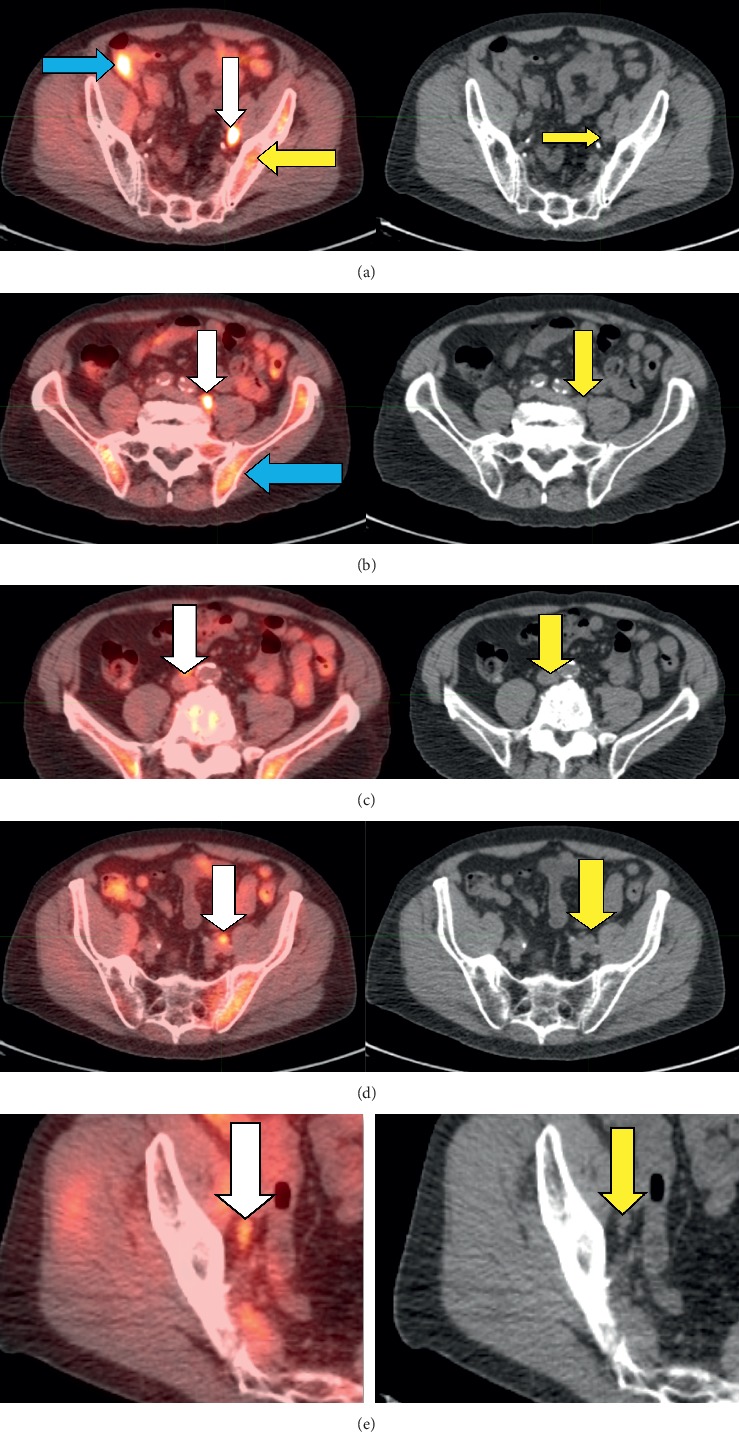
Images of a 68-year-old male who had undergone radiation therapy, followed by short-term androgen deprivation therapy. He was referred for ^18^F-fluciclovine imaging, on no treatment, when his PSA rose to 10.5 ng/mL. Five rows of images from his study are shown, to demonstrate varying intensities of uptake. SUVs, a semiquantitative measure of uptake intensity, are given, with higher values denoting greater intensity; compare to marrow uptake at the same level (yellow arrows in PET images). First (top) row: the first, left-hand image identifies a 1.4-cm left obturator node (SUV: 7.6) (white arrow). The blue arrow points to physiologic bowel uptake. The yellow arrow points to physiologic uptake in the marrow of the left iliac bone. The image on the right is the corresponding CT view; the yellow arrow points to the left obturator node. Second row down: left-hand image shows metastasis at the level of the common iliac nodes (SUV: 5.8). The blue arrow points to typical, heterogeneous bone marrow uptake (normal). The corresponding CT image on the right shows the node adjacent to the psoas muscle. Third row down: the left-hand image identifies a 1-cm aortocaval metastasis (SUV: 3.2). The yellow arrow on the corresponding CT view points to a lymph node at this site. Fourth row down: the left-hand image identifies a 1-cm left external iliac node (SUV: 3.5). The yellow arrow on the right points to the node on the CT view. Fifth (bottom) row: the left-hand image shows a 6-mm right obturator node (white arrow). Although the SUV is only 2.7, the node still clearly shows enhanced uptake compared to the adjacent iliac marrow. CT image on the right identifies the node (yellow arrow).
